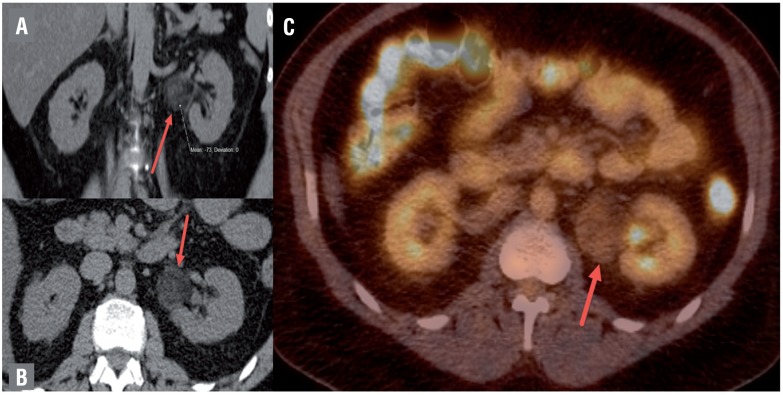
CT Images. a) Coronal non-contrast CT demonstrating a peri-renal mass with heterogeneous attenuation. Hounsfield units measured at −73 indicating fat in mass. b) Axial non-contrast CT again demonstrating left perirenal mass with heterogeneous attenuation arising from retroperitoneum. c) Axial PET/CT shows mild increased FDG uptake with SUV (standardized uptake value) measurements of up to 2.8, which could represent malignancy or inflammatory process.
